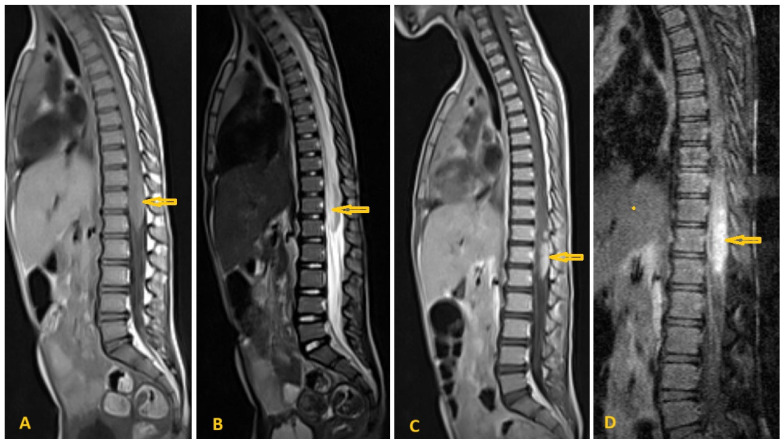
Selected sagittal images of lumbar spine MRI of a 5-year-old girl who presented with lower limb weakness and urine retention for a week and who had a history of excessive swimming in a valley during a journey two months before. The selected images show isointense expansion of the distal spinal cord and conus medullaris (arrows) in the T1-weighted image (A), with patchy hyperintense foci in T2-weighted images (B), intramedullary peripheral nodular enhancement in Gadolinium-enhanced T1-weighted images (C), and intramedullary increased signal intensity in the fat-suppression image (D). MRI revealed no extension into the neural foramina and no bone or surrounding soft tissue edema. The whole picture was consistent with inflammatory spinal Schistosomiasis.
